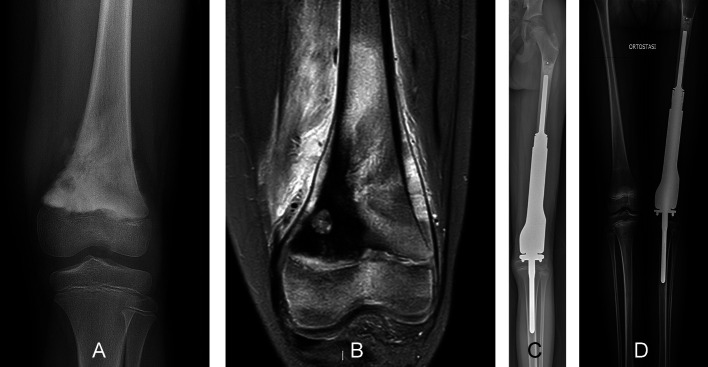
A 10-year-old boy, diagnosed with osteosarcoma of the left distal femur, who was treated by osteoarticular resection followed by reconstruction using an expandable modular prosthesis. (A) An anteroposterior radiographic view showed osteosarcoma of the distal femur infiltrating the growth plate. (B) Coronal-fat saturation magnetic resonance image showing the location of the tumor at the distal femur. (C) Postoperative radiographs showing reconstruction of the distal femur with an expandable modular prosthesis at the 5-month follow-up. (D) Radiograph of the lower limbs showing elongation of the modular prosthesis at the 2-year follow-up. The extendable prosthesis was able to avoid discrepancies in children undergoing resection of the distal femur with sacrifice of the growth plate.
